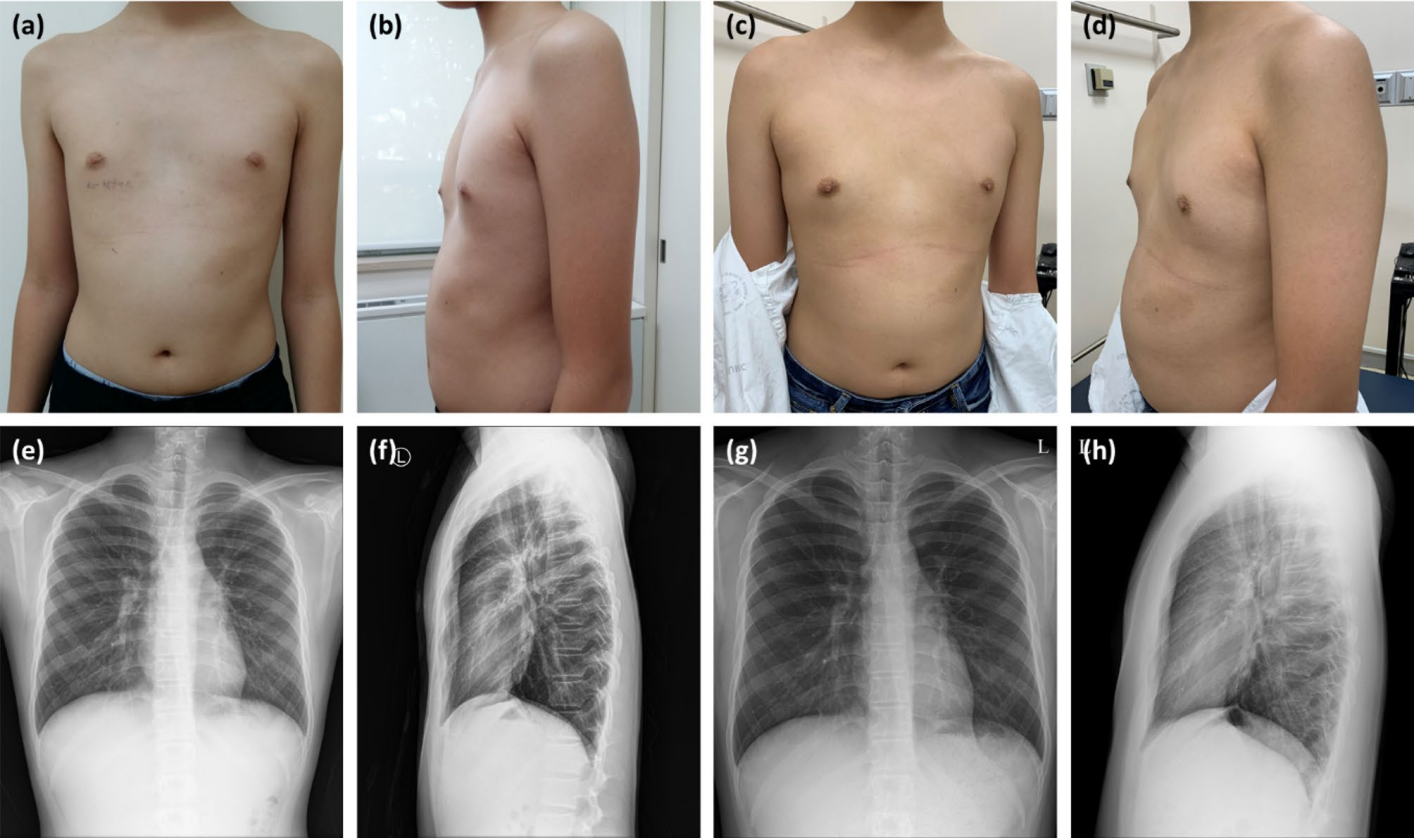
Evaluation of treatment outcomes after 1 year of vacuum bell (VB) application. Pictures and chest radiographs were taken from a 17-year-old male patient just before starting treatment (a, b, e, f) and at 1 year after VB therapy (c, d, g, and h). (a) Anterior view before VB application; (b) lateral view before VB application; (c) anterior view at 1 year after treatment; (d) lateral view at 1 year after treatment; (e) anteroposterior view on chest radiographs taken before starting therapy; (f) lateral view before starting therapy; (g) anterolateral view after 1 year of treatment; and (h) Lateral view after 1 year of treatment.
